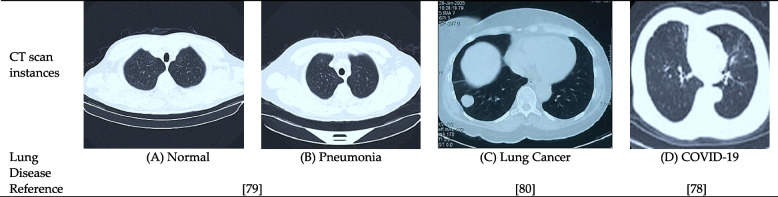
Instances of CT scans for prominent lung diseases
